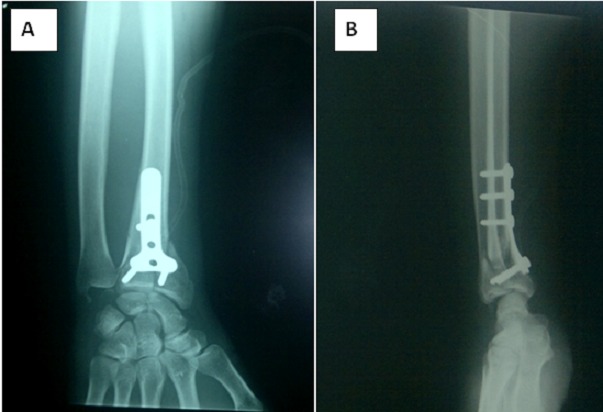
(A) anteroposterior radiograph of the left wrist joint immediately after surgery: the fractured distal end of the radius fixed with a T-volar plate fixation; (B) postoperative lateral radiograph of the left wrist
